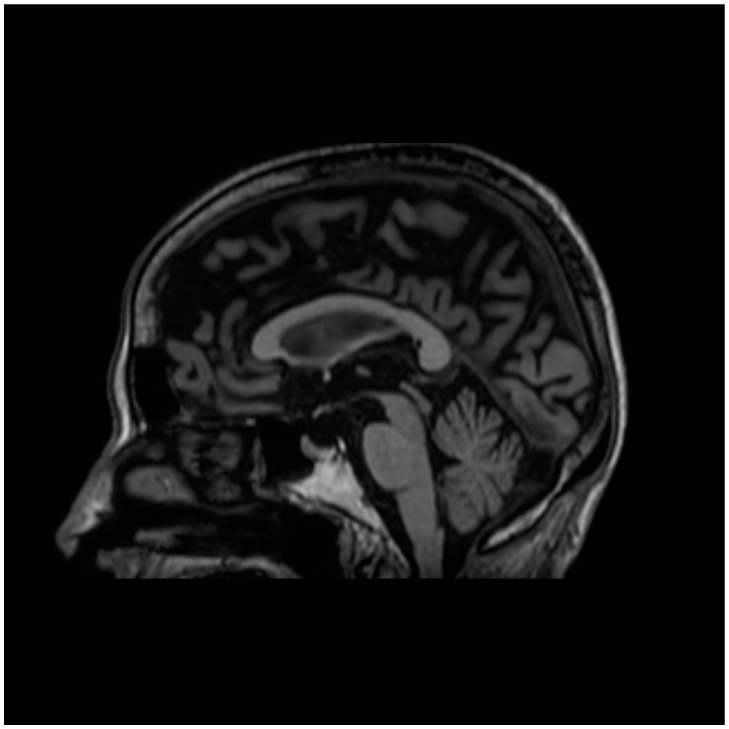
Sagittal MRI T1 scan demonstrating characteristic hummingbird sign in PSP.
